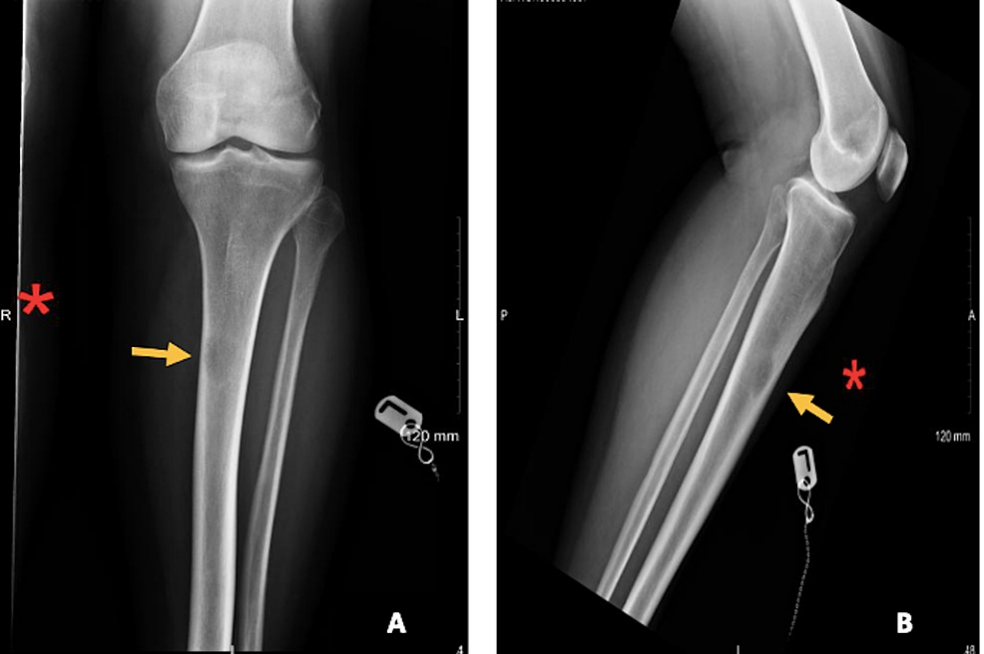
Left tibia/fibula radiography (A) Anteroposterior view of the left tibia/fibula radiography and (B) lateral view of the left tibia/fibula radiography show focal intramedullary lytic lesion at the proximal left tibia (arrow) suggestive of bone tumour.
